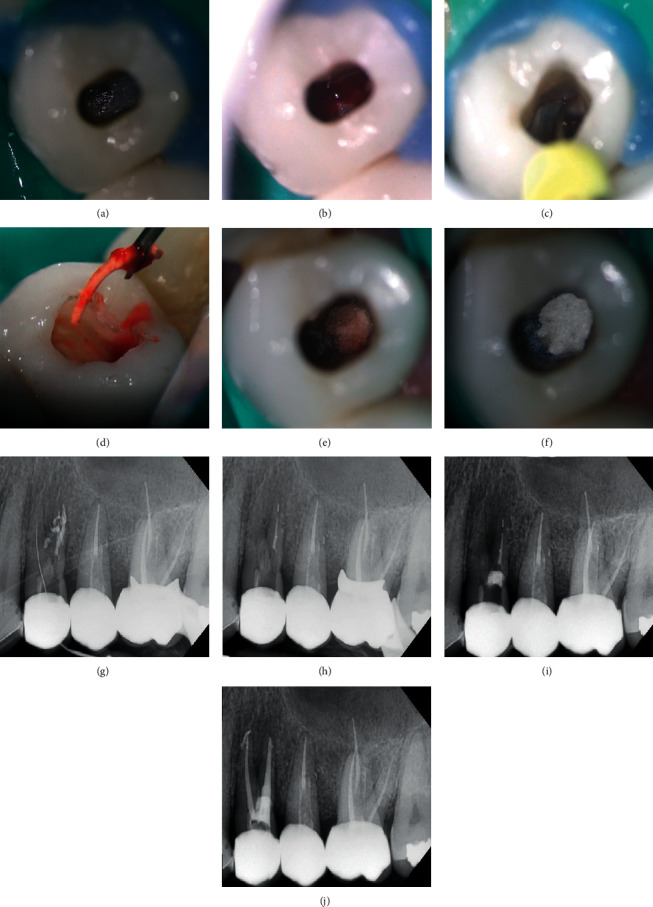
Clinical images and periapical radiographs of the retreatment of tooth #24. (a) Access cavity; (b) bleeding perforation; (c) exploring the palatal canal; (d) gutta-percha removal from the perforation; (e) collagen sponge placement; (f) 5MO cement application; (g) radiography of palatal canal localization; (h) cleaned perforation site; (i) radiography of 5MO cement application; and (j) radiography of obturation.
